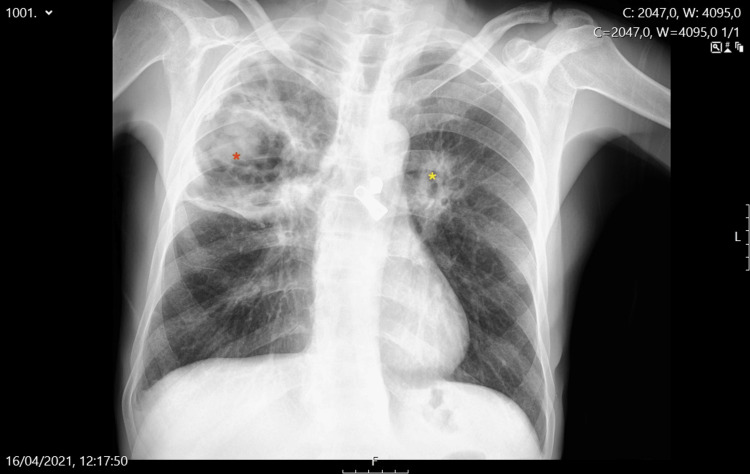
Chest radiograph at hospital admission showing cavitary lesions The chest radiograph taken on April 16, 2021, demonstrated a large cavitary lesion in the right upper lobe (red asterisk) and a smaller cavitary lesion in the left lung (yellow asterisk). These findings were later associated with the diagnosis of granulomatosis with polyangiitis (GPA)
